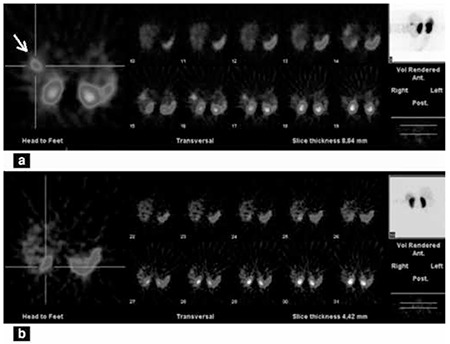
In-111 octreotide SPECT image at 24 hours showing activity in the liver on axial slice (a), 72 hours SPECT image showing clearance of the uptake (b)
